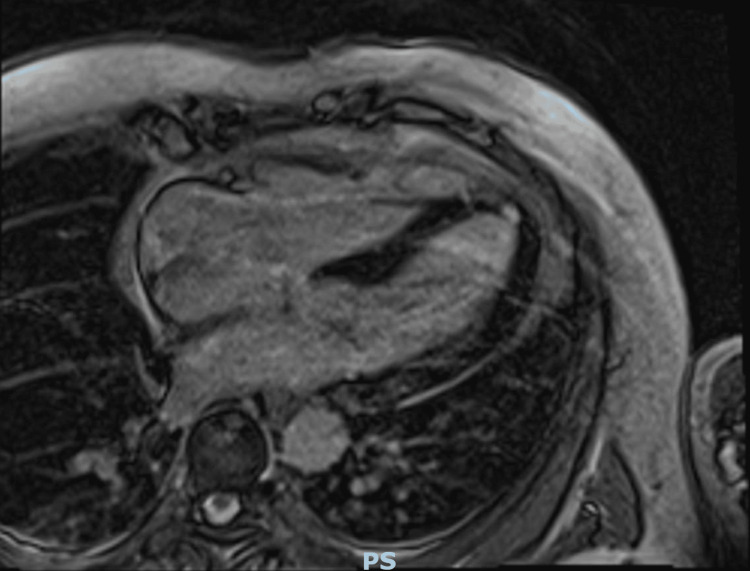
Cardiac MRI showing no evidence of late gadolinium enhancement
